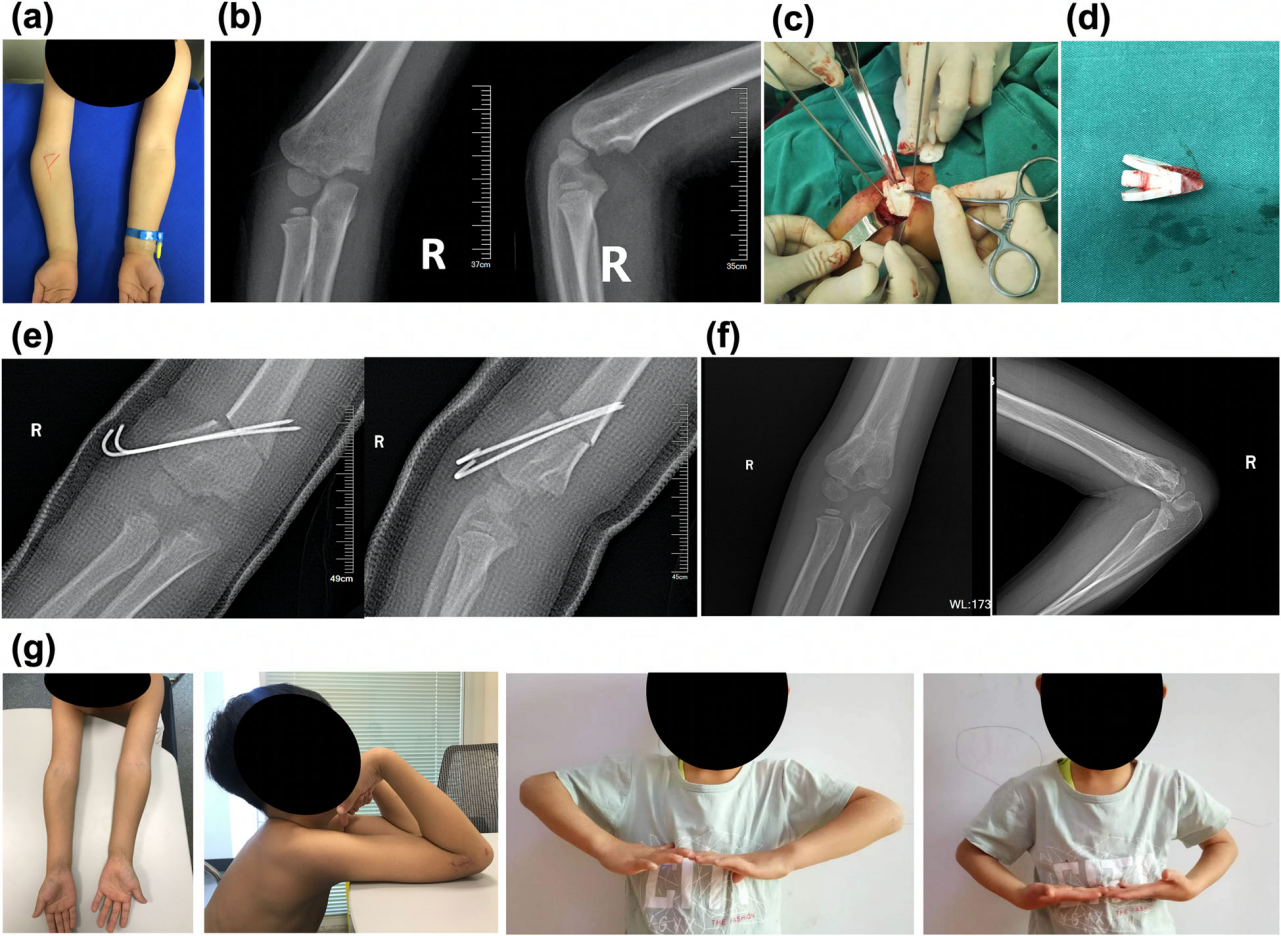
Radiographs and comparison between preoperative and intraoperative appearance. a Appearance showing different carrying angle of bilateral upper limbs and varus deformity of the right elbow. b Posteroanterior radiograph of right elbow joint showing cubitus varus deformity before operation. c Individual osteotomy navigation template matched the distal humerus well, and fixation of the navigate template with one Kirschner wire; two holes were drilled with the Kirschner wire to control rotational rectification. d Osteotomy was performed according to the navigation template surface, and the osteotomy block was completely consistent with the preoperative design. e Lateral radiograph of right elbow joint showing no extension or flexion deformity after operation. f Postoperative appearance showing correction of the deformity 10 weeks after operation. g Appearance showing correction of the deformity after operation, with no limit to the flexion and extension of the elbow joint and rotation of the forearm
